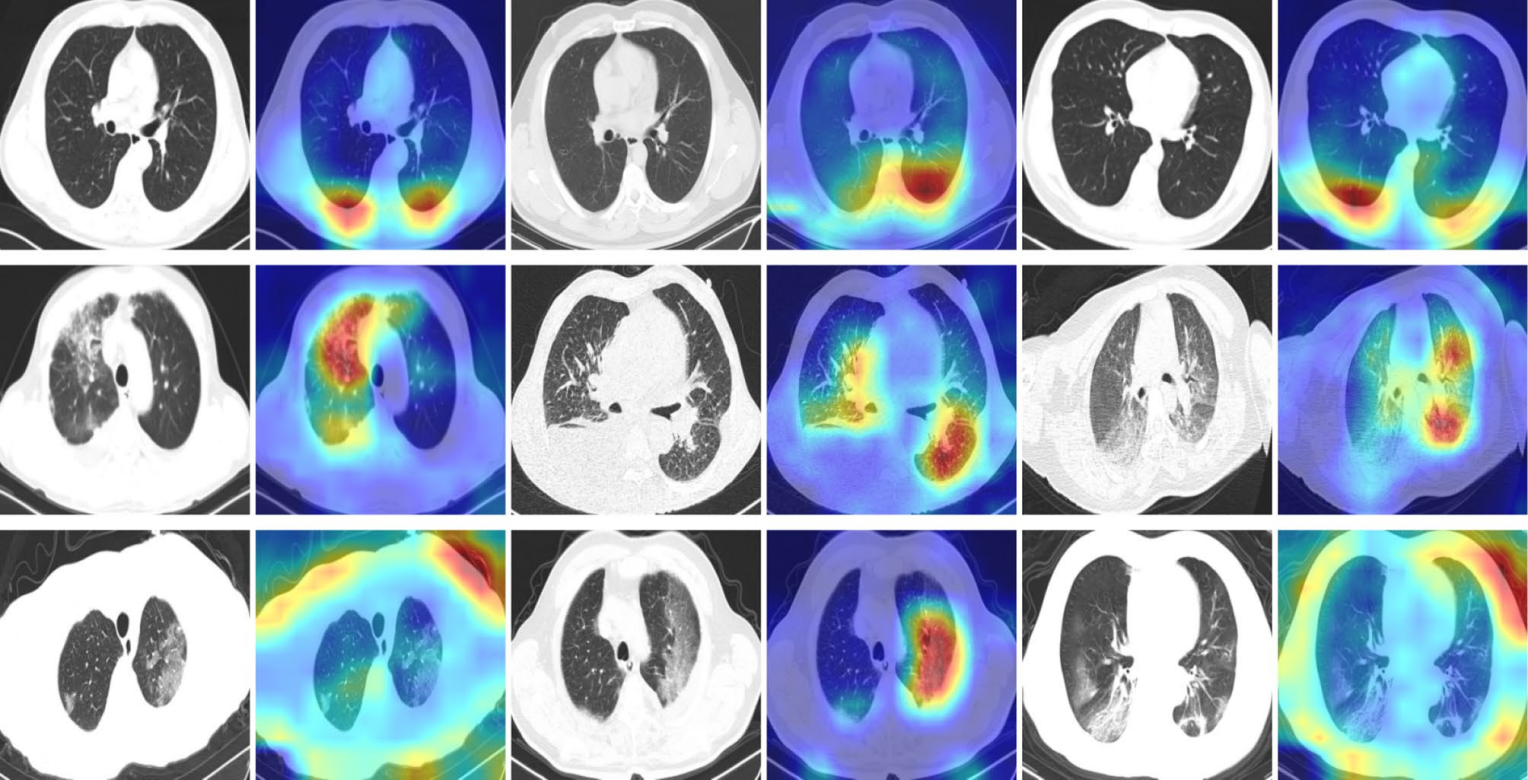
Grad-CAM visualization of Bit-M. The first line depicts the normal case, the second line the case of CP, and the third line the case of COVID-19 (NCP) due to infection by SARS-CoV-2. The model using CT images as input source have yielded accurate predictions.
